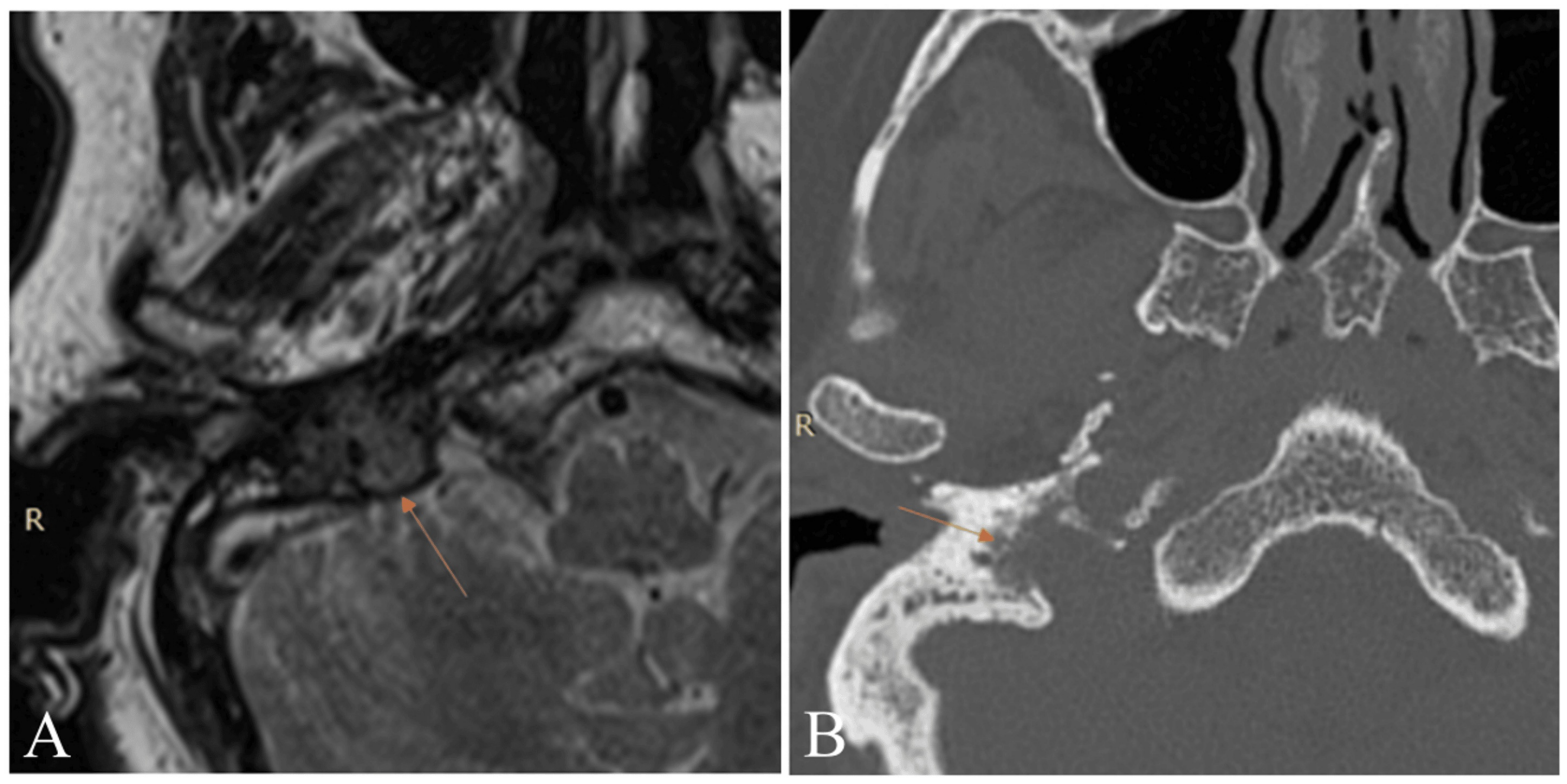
Imaging of case 8. (A) T2-weighted MRI (arrow) showing a vascular tumor in the right jugular foramen with flow voids (salt and pepper sign). (B) High resolution axial CT of the internal auditory canal (IAC) without contrast showing the tumor eroding the jugular foramen walls.
